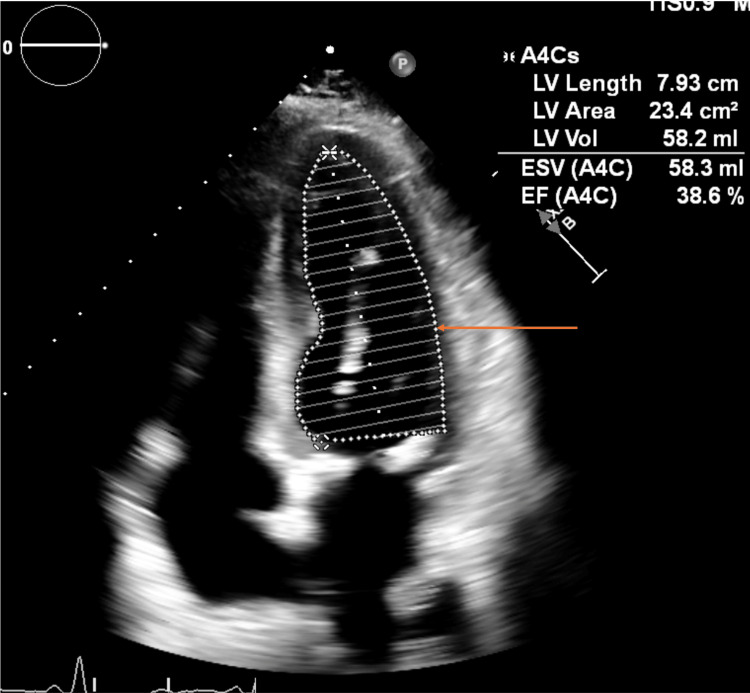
Apical four-chamber view of the heart on echocardiogram, with left ventricular (arrow) ejection fraction being calculated.
